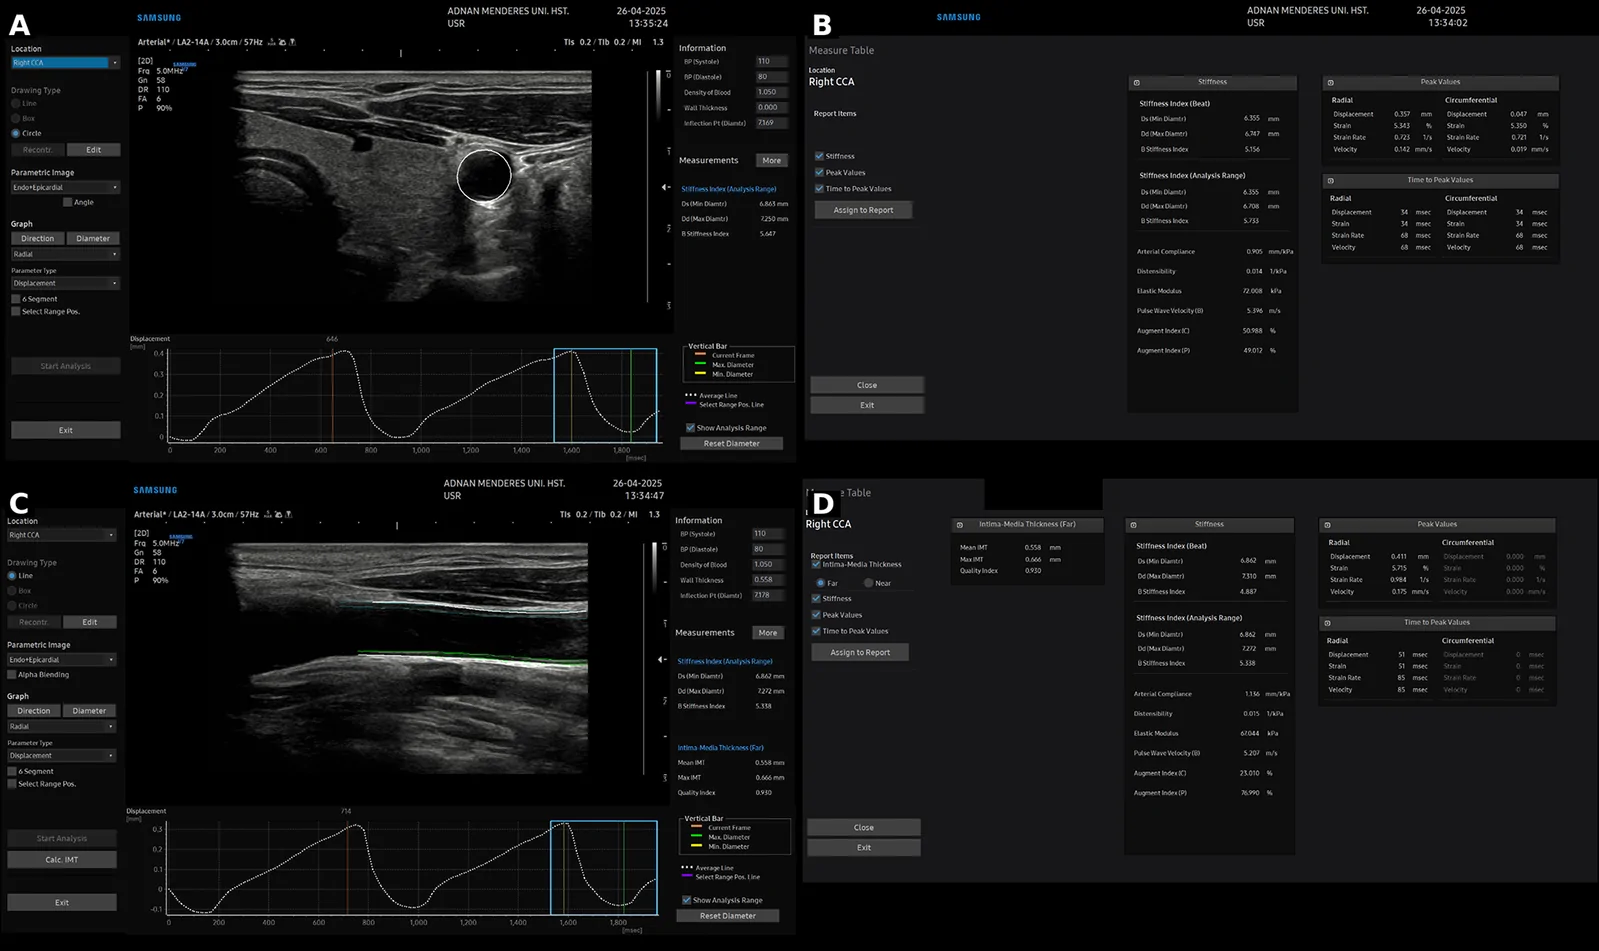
Representative STCS-US examination in a control participant. (A) Transverse-plane carotid ultrasound image with speckle-tracking analysis. (B) Transverse-plane stiffness and strain measurements generated by the Arterial Analysis software program. (C) Longitudinal-plane carotid ultrasound image with speckle-tracking analysis. (D) Longitudinal-plane stiffness and strain measurements generated by the Arterial Analysis software program
